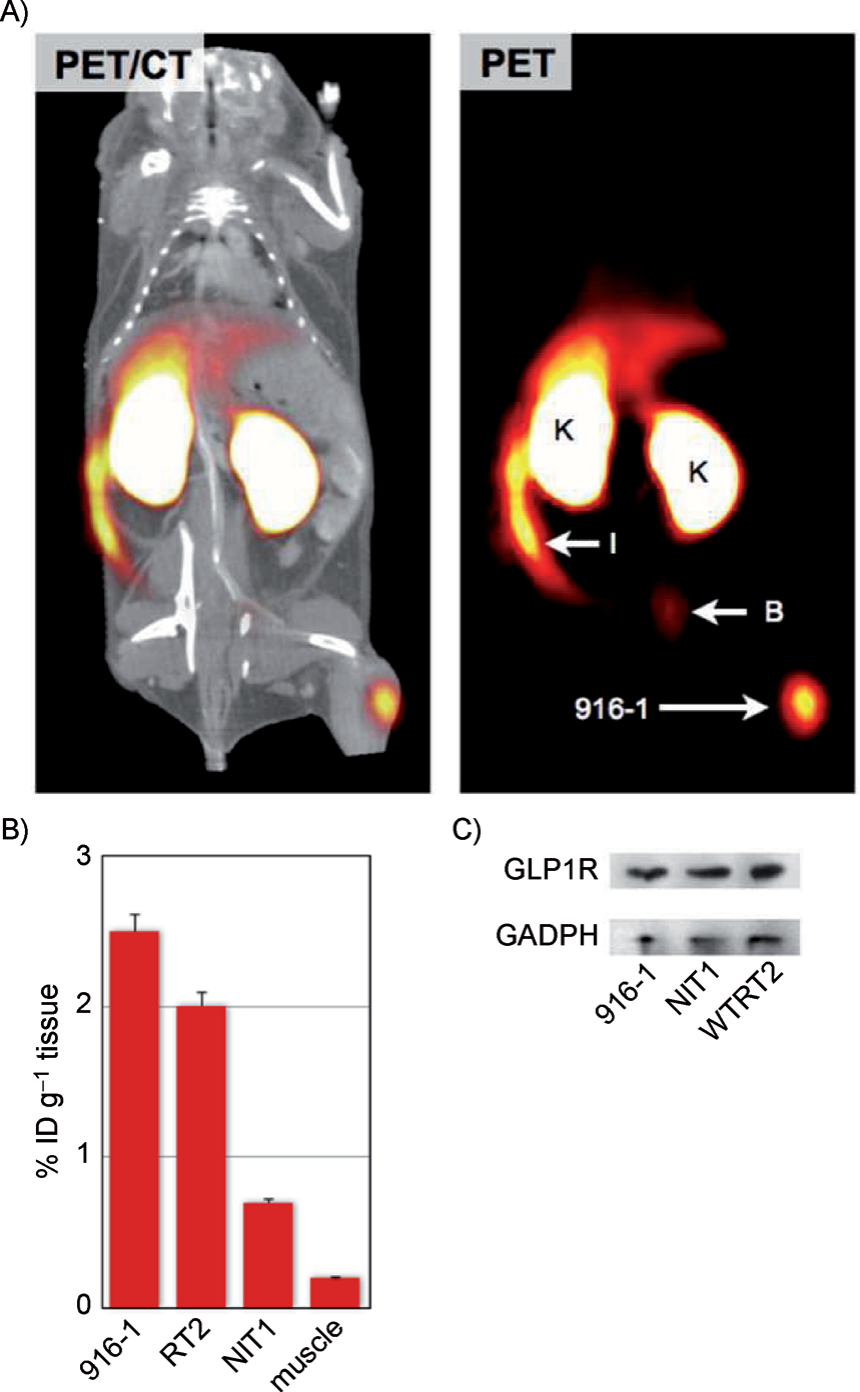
In vivo imaging of insulinoma. A) PET-CT and PET only scans of ^18^F-E4_Tz12_
